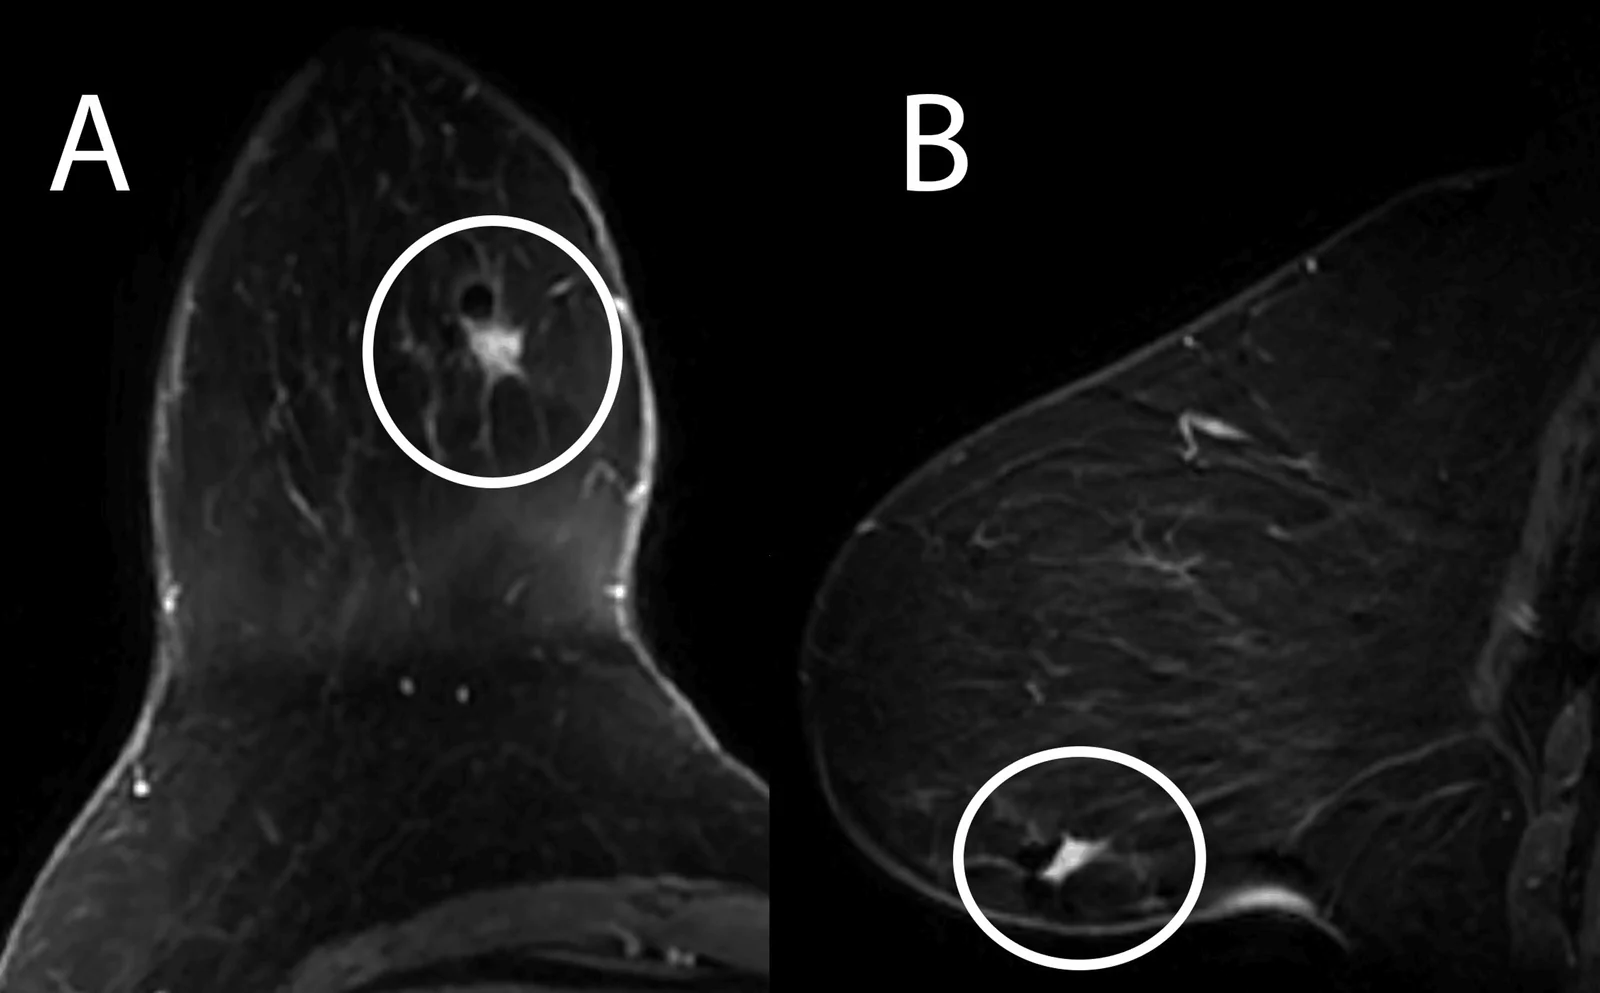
Preoperative breast MRI performed for extent of disease. (A) Axial and (B) sagittal MR images of the right breast, after percutaneous biopsy diagnosed fibromatosis-like metaplastic triple negative breast cancer, redemonstrate the malignancy as an irregular mass with spiculations (open white circle). There was no evidence of multicentric disease, multifocal disease, or contralateral synchronous malignancy.
